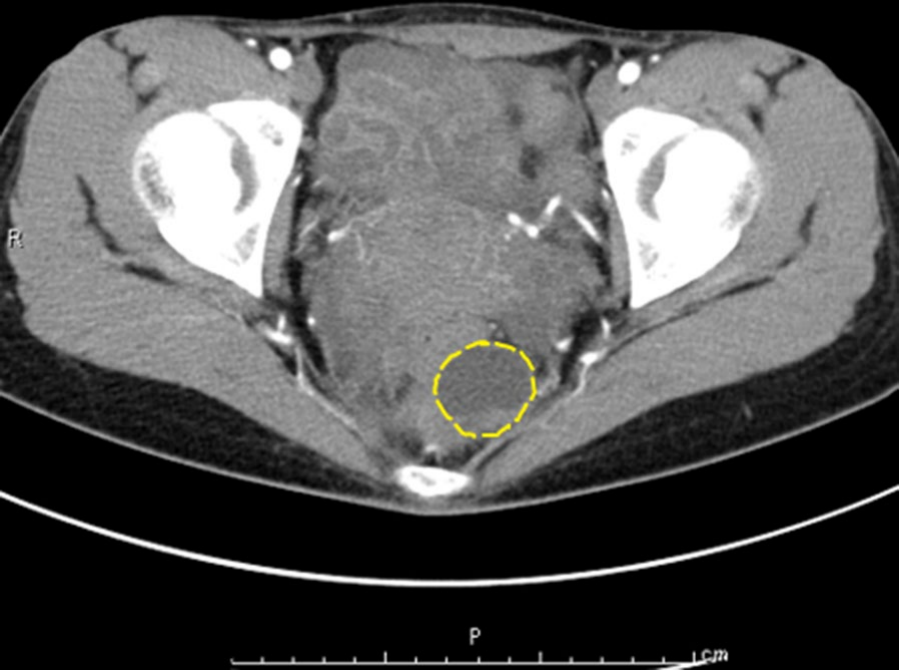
CT showed a multilocular cystic mass on the dorsal side of the rectum in front of the sacrum (dotted line)
